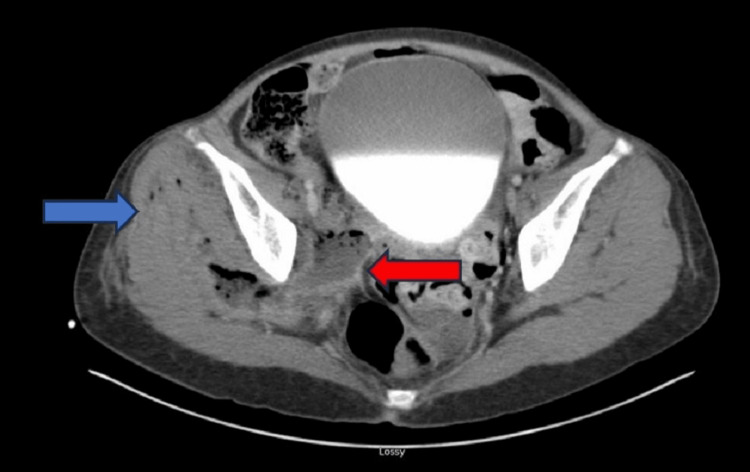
Axial CT image of the pelvis demonstrating a complex pelvic abscess characterized by fluid and gas collections in the right hemipelvis (blue arrow), extending into the gluteal musculature. Notably, the abscess shows a clear communication with an adjacent loop of small bowel (red arrow) CT: computed tomography
